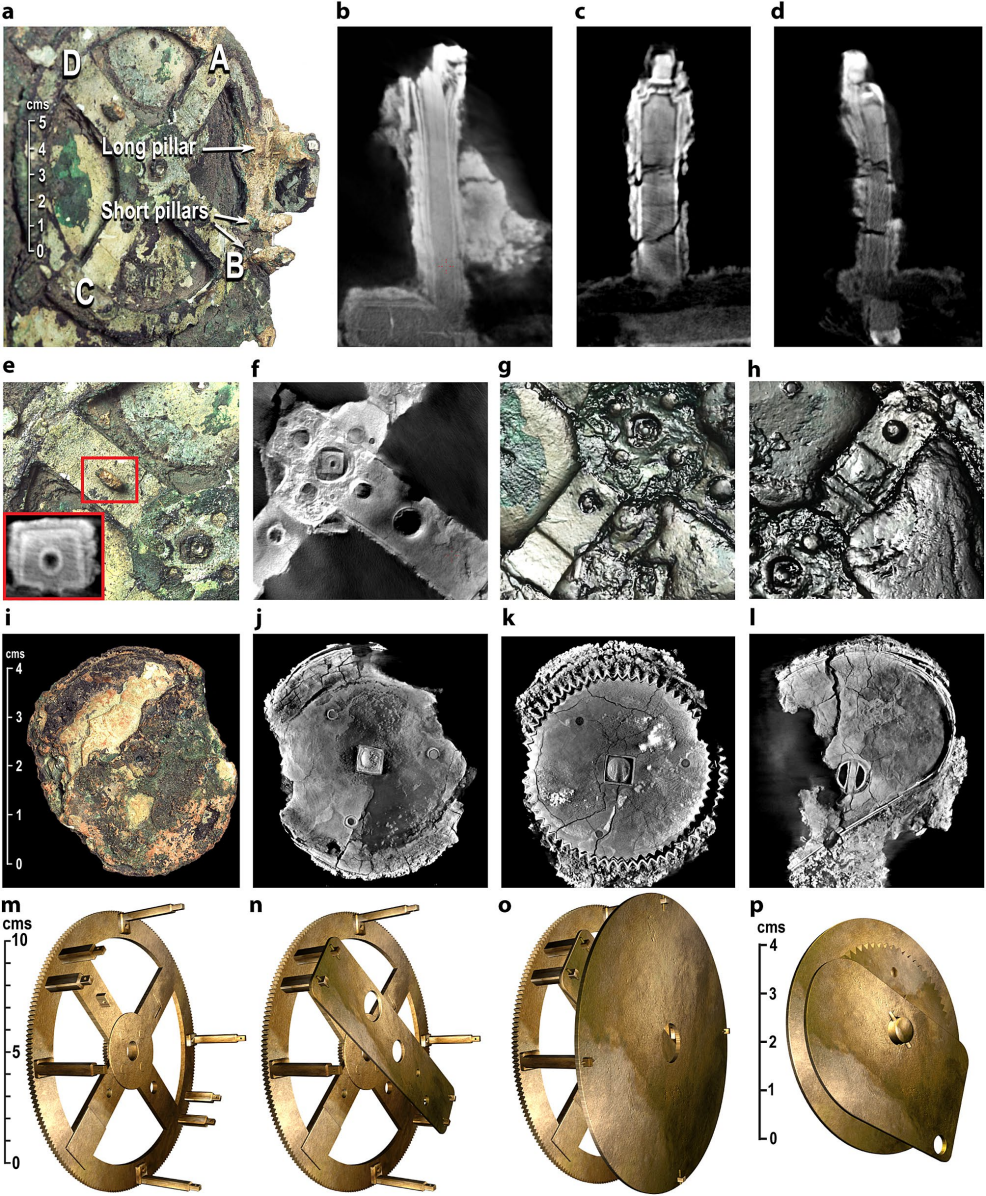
Evidence from Fragments A & D. Reconstructed plates and gear. (a) Photograph of Fragment A, showing pillars on the periphery of b1 and features on Spokes A, B, C, D. (b) X-ray CT of long pillar. (c–d) X-ray CT of short pillars. (b–d) are from an improved X-ray volume^22^. All pillars have shoulders and pierced ends. (e–h) Photographs, PTMs, X-ray CT: features on Spokes D, B, C, A, including holes, circular depressions and flattened areas. In (E), the pierced block on Spoke D is highlighted in red, with inset showing X-ray CT slice through the block. (i-l) Photograph & X-ray CT of Fragment D, showing a *disk*, *gear* and *plate*. (m–o), Computer reconstruction, showing b1, Strap on the short pillars; Circular Plate (CP) on the long pillars. (p) Computer reconstruction of the features in Fragment D, which we reconstruct as the epicyclic components of a Venus mechanism.
